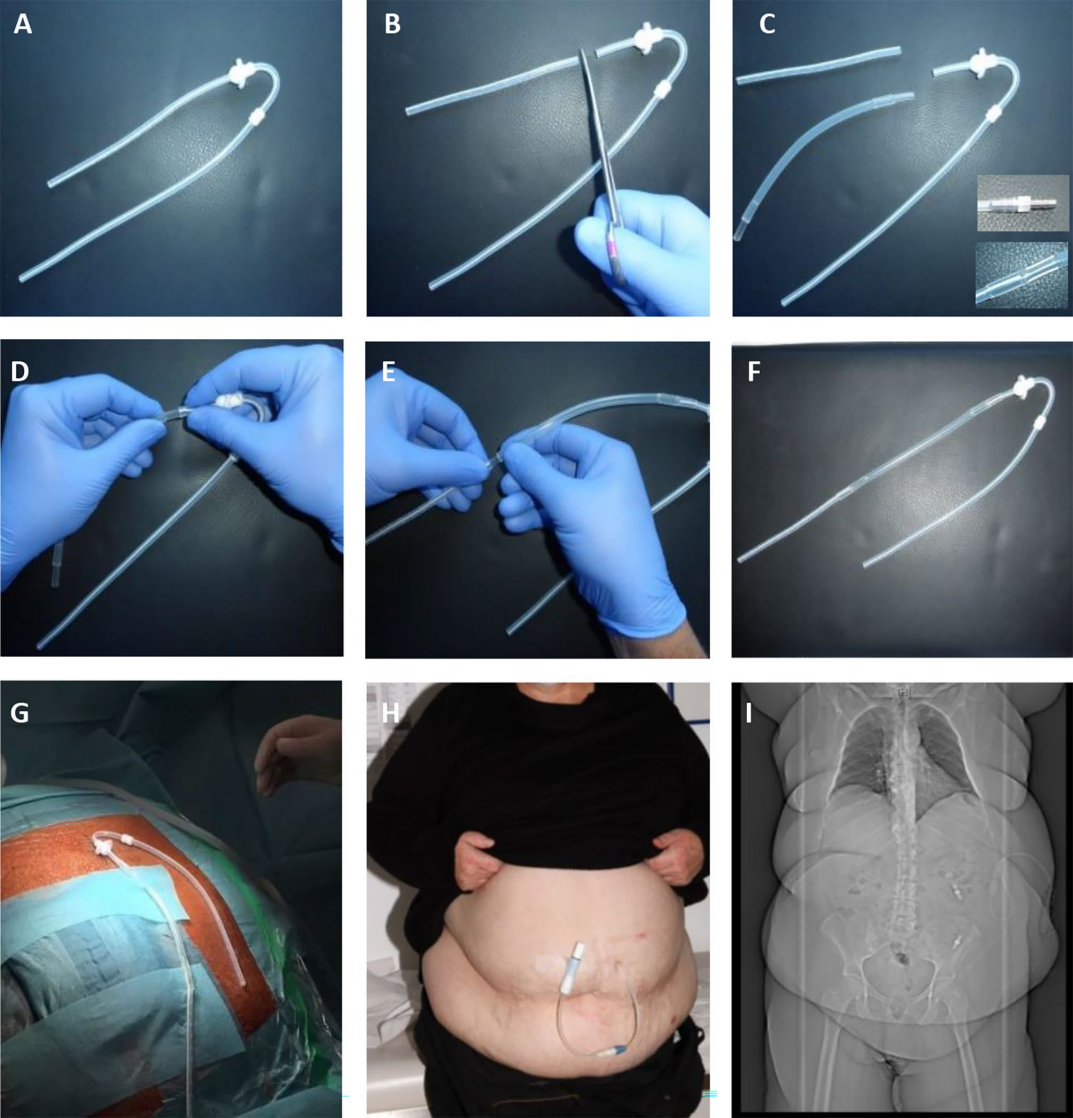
Assembly of an extended swan neck PD catheter and position of the PD catheter in a representative patient with obesity (BMI 47 kg/m^2^). After cutting off the intraperitoneal segment of the swan neck PD catheter (A, B), two titan (Covidien 8888–415,612) or silicon adaptors (Oriplast, Neunkirchen, Germany REF 260.480) were mounted on a flexible silicon rubber tube that was adjusted to the patient’s figure and connected to the proximal end (C–E). Finally, the extended catheter was completed using the intraperitoneal segment that had previously been cut off (F). These steps were performed in the OR under sterile conditions. Intraoperatively, the implantation position in the upper abdomen was adjusted according to the patient’s figure (H). Position of the catheter after implantation with the patient standing (H) or lying for CT (I), which was performed for a different reason. Note the two titan adaptors in the left upper and lower abdomen
